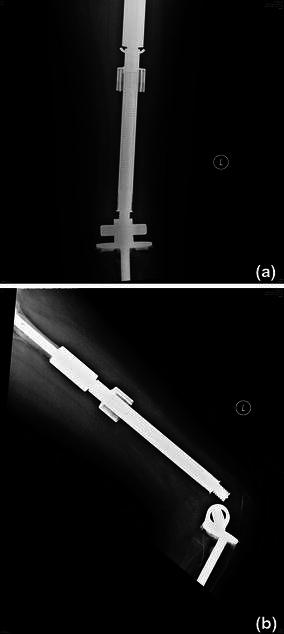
Anteroposterior (a) and lateral (b) radiographs of a pediatric patient with a REPIPHYSIS endoprosthesis
